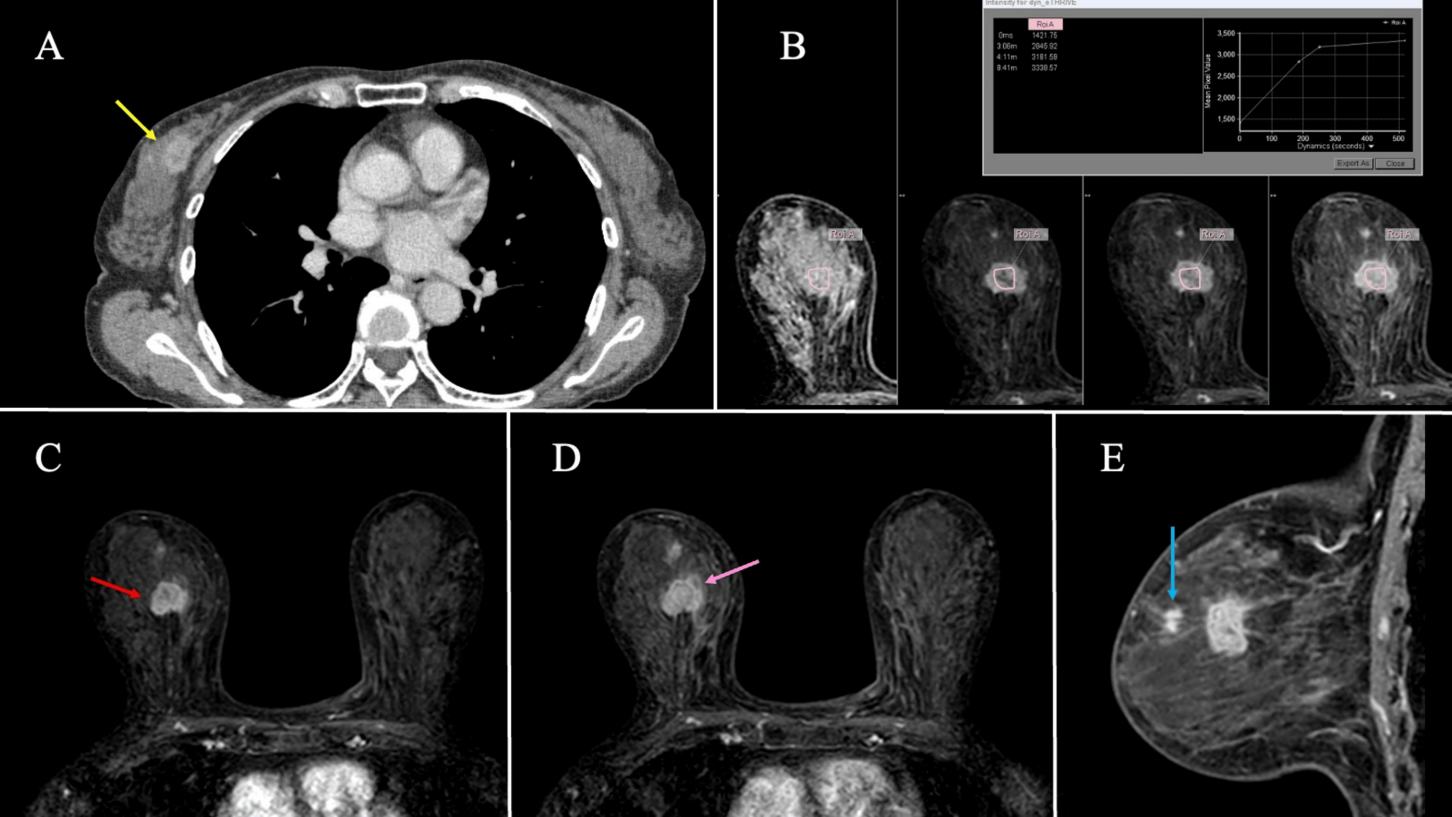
Contrast-enhanced CT and MRI findings. (A) Contrast-enhanced CT demonstrating a dumbbell-shaped mass with variable contrast enhancement in each component (yellow arrow). (B-D) MRI in the axial view: (B) time-intensity curve demonstrating a fast-to-plateau pattern; (C) early strong enhancement in the lateral portion of the mass (red arrow); (D) progressive delayed enhancement in the medial portion (pink arrow). (E) MRI in the sagittal view also depicts a focal lesion toward the nipple, suggestive of a possible daughter lesion (blue arrow).
